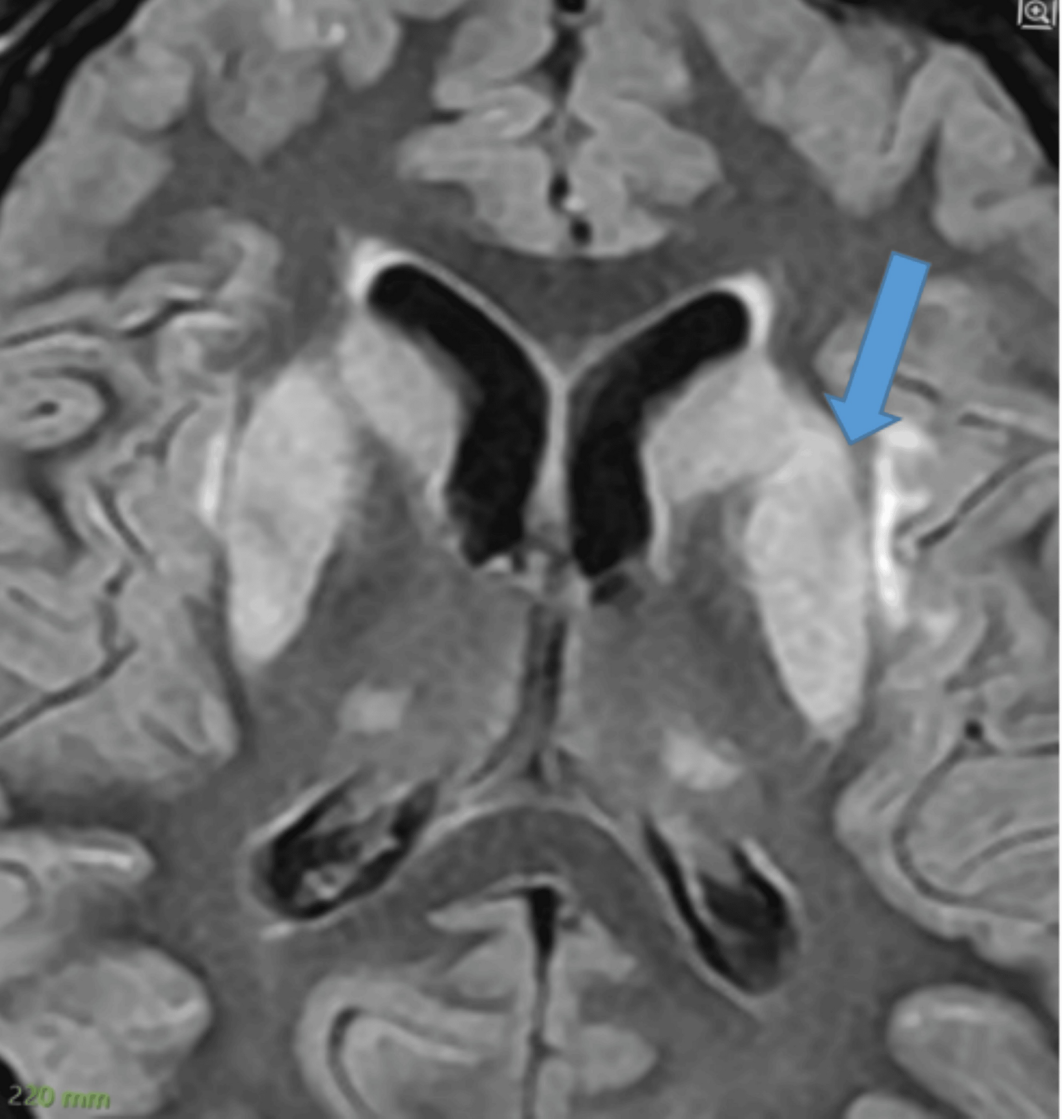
FLAIR Axial MRI Sequence Showing Hyperintense Signal (Blue Arrow). FLAIR: fluid-attenuated inversion recovery; MRI: magnetic resonance imaging
